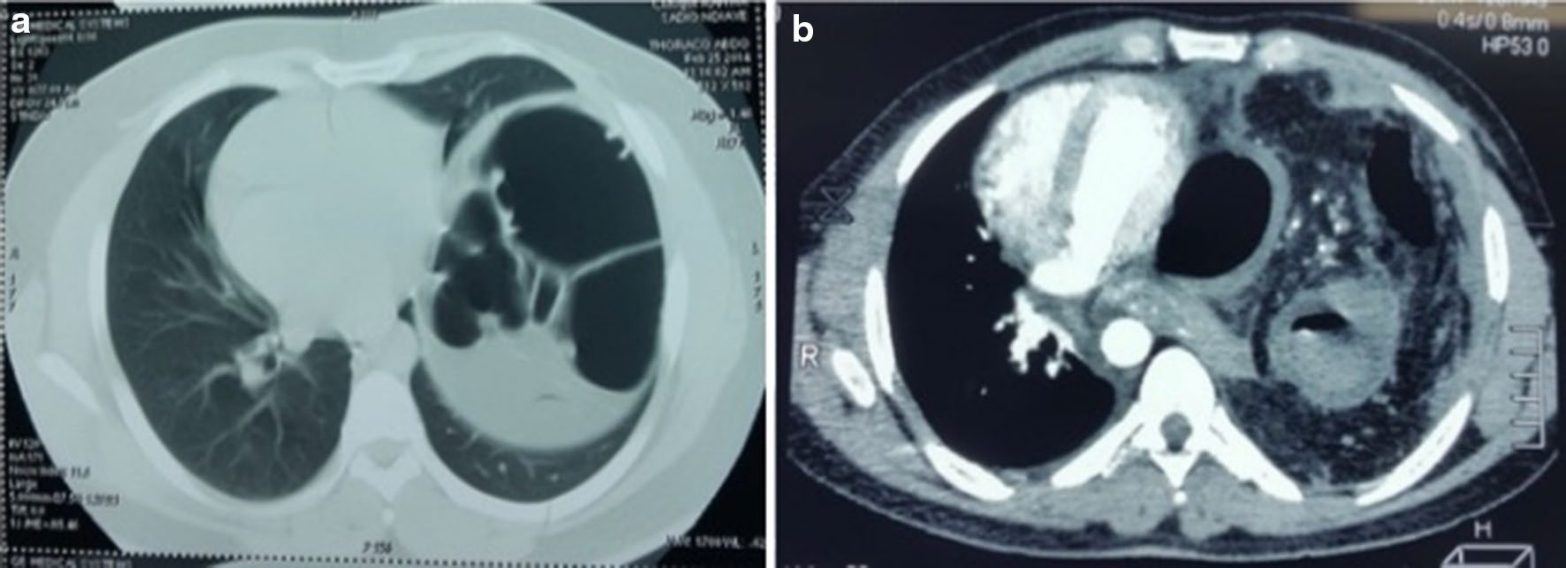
a, b Chest CT scan showing a left diaphragmatic hernia
